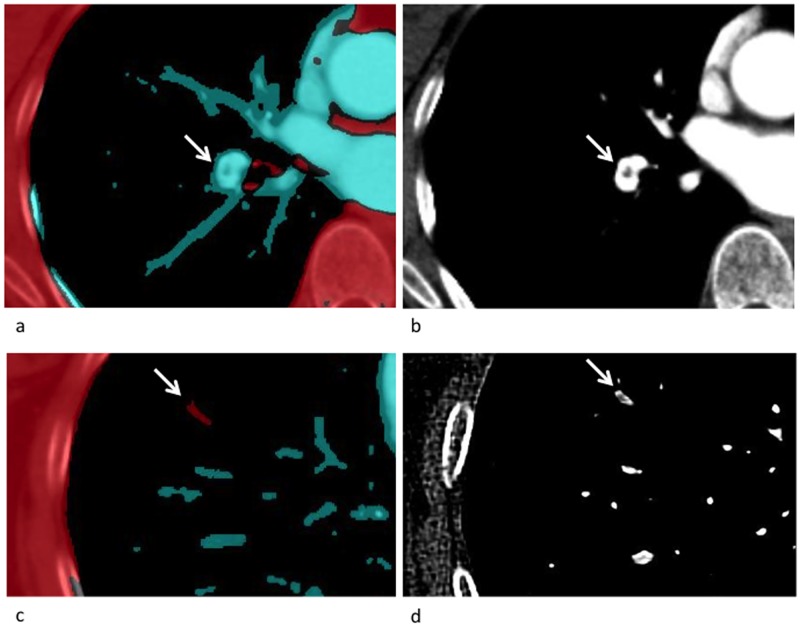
Grey scale CT and DECT vessel reconstruction from a female patient (75y) diagnosed with stage IV melanoma. A proximal embolus in right lower lobe was not highlighted by the DECT vessel reconstruction software (a) but reliably diagnosed on grey scale CT (b). A subsegmental embolus in right lower lobe was primarily detected in DECT vessel reconstruction (c), and could retrospectively be delineated in thin-slice (1 mm) reconstructions of the corresponding grey scale CT (d).
